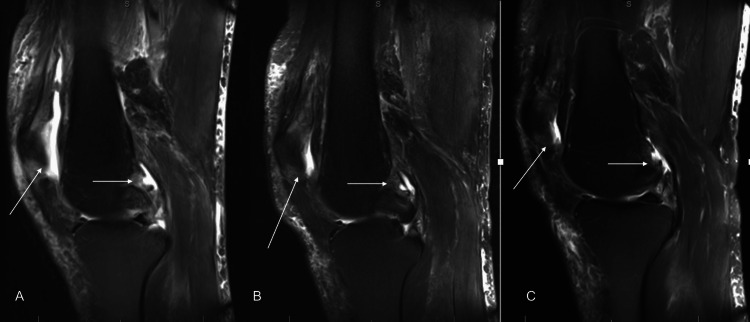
Serial postoperative MRI MRI taken at six weeks postoperatively (A), six months postoperatively (B), and one year postoperatively (C). The arrows represent areas of effusion and the anterior knee arrow also points to anterior synovitis.
